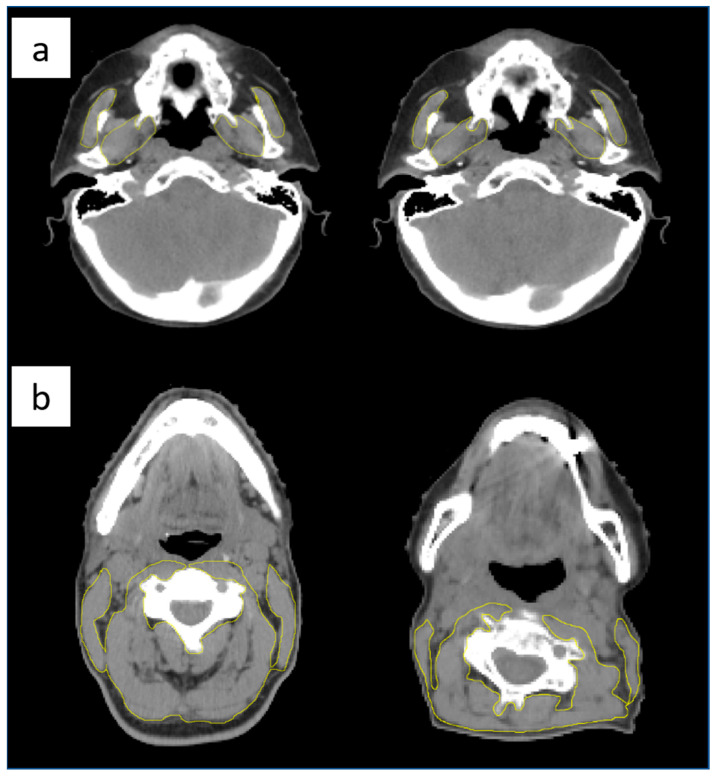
Axial CT-scan image delineation of the masticatory muscles (a) and paravertebral and sternocleidomastoid muscles at the level of the third cervical vertebra (b) in a non-sarcopenic patient (left) and in a sarcopenic patient (right).
